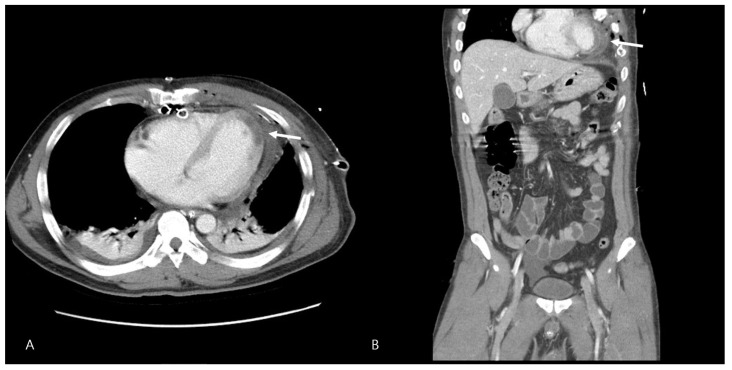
Essential images of chest CT findings. Postoperative day 1 contrast-enhanced chest CT. Axial (A) and coronal reformatted (B) images show a focal hypoattenuating area in the anterior/anterolateral wall of the left ventricle (arrows), consistent with myocardial contusion.
